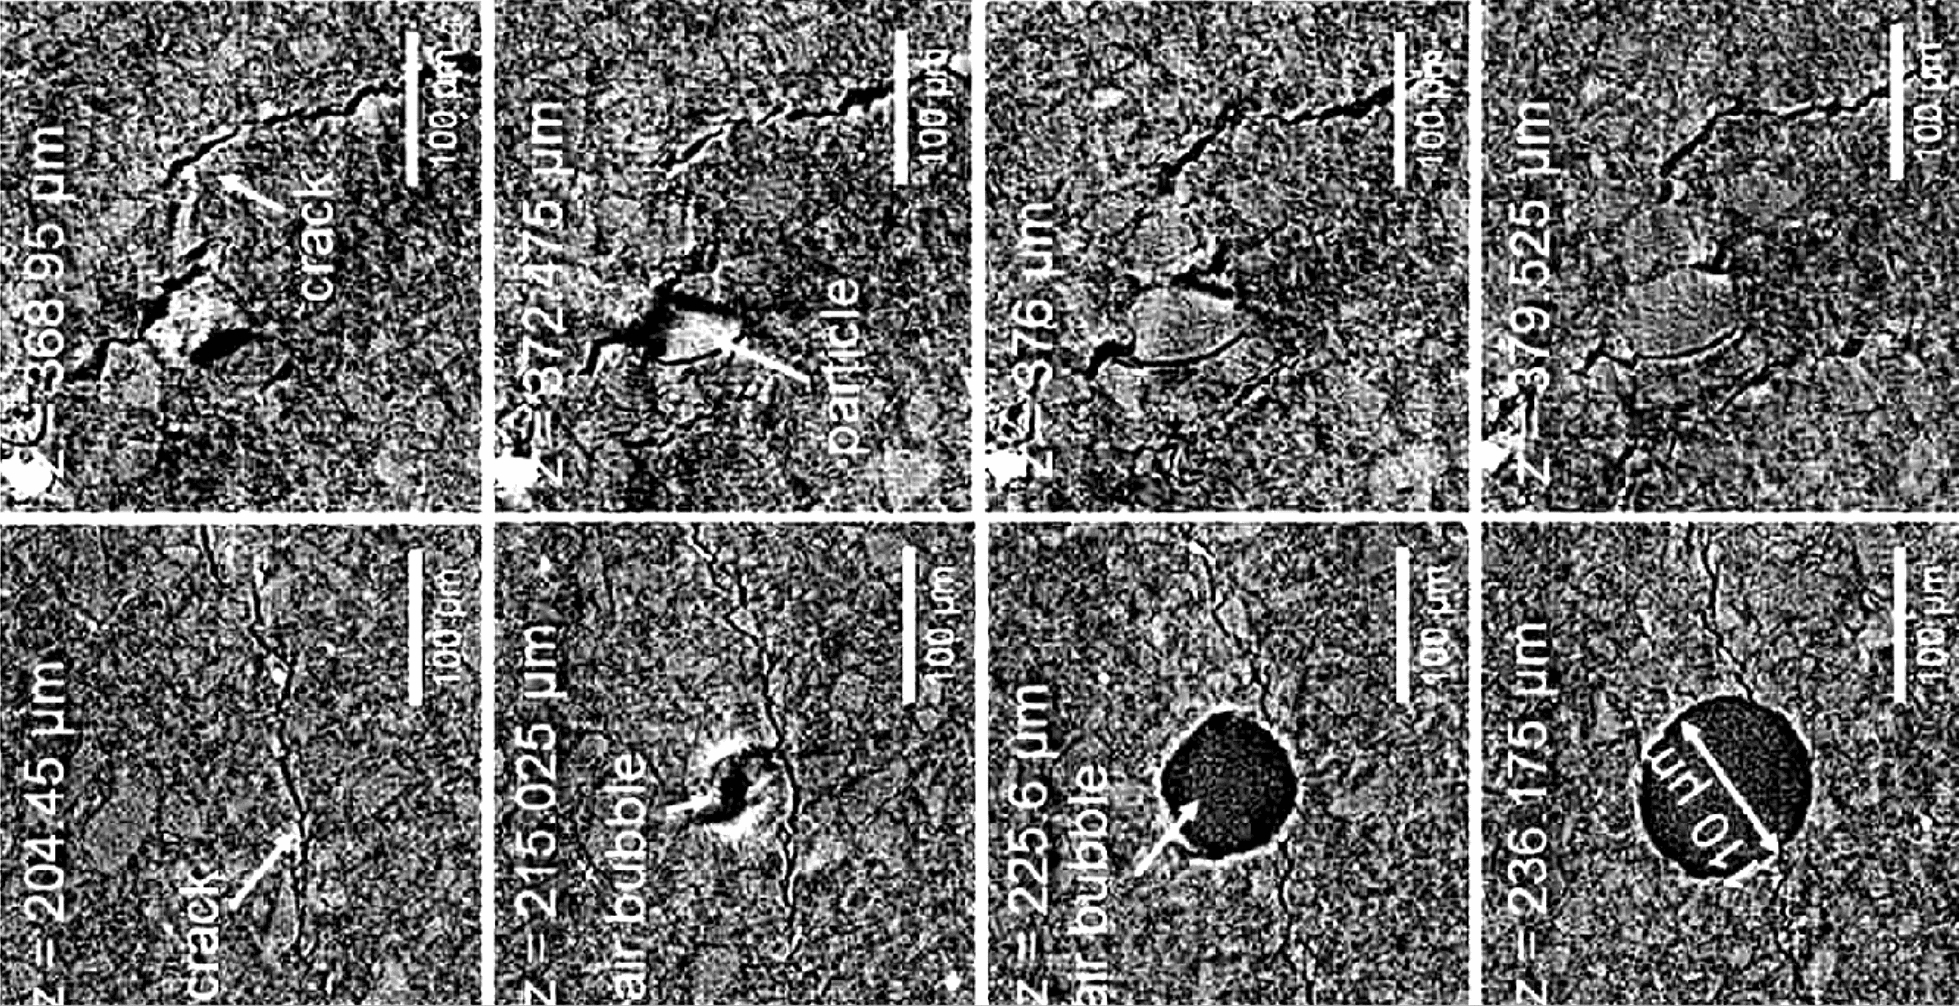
Synchrotron X-ray image slices of different layers showing voids and cracks in chocolate samples [48]
